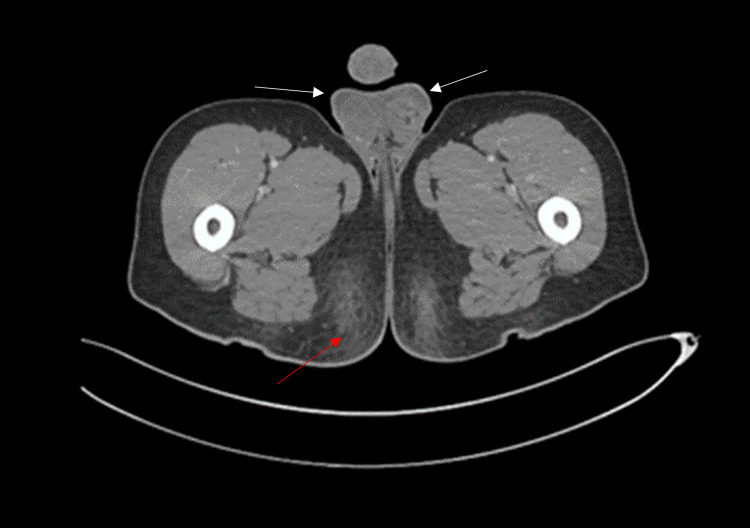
Axial view of computed tomography of the pelvis with IV contrast demonstrating diffuse subcutaneous edema with scrotal wall thickening bilaterally. No soft tissue/fat stranding, fascial thickening nor free gas was noted (red arrow: diffuse subcutaneous edema; white arrow: scrotal wall thickening bilaterally).
